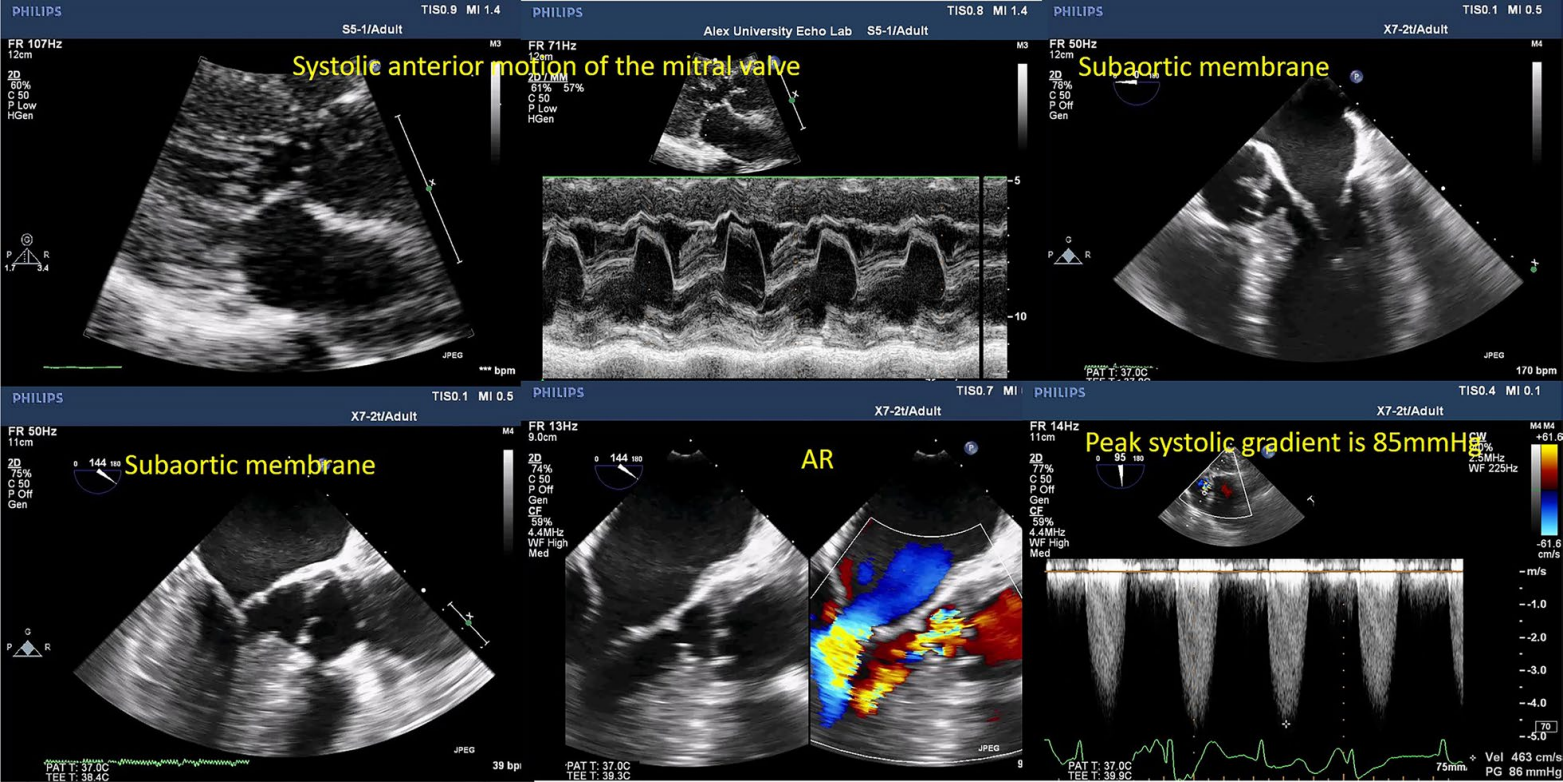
A right upper and middle panels, parasternal long-axis view, and M-mode show left ventricular hypertrophy and systolic anterior motion of the mitral valve. 2D TOE (left upper and right lower and middle panels) shows a subaortic membrane and aortic valve regurgitation. In the left lower panel, high peak and mean transaortic gradients
